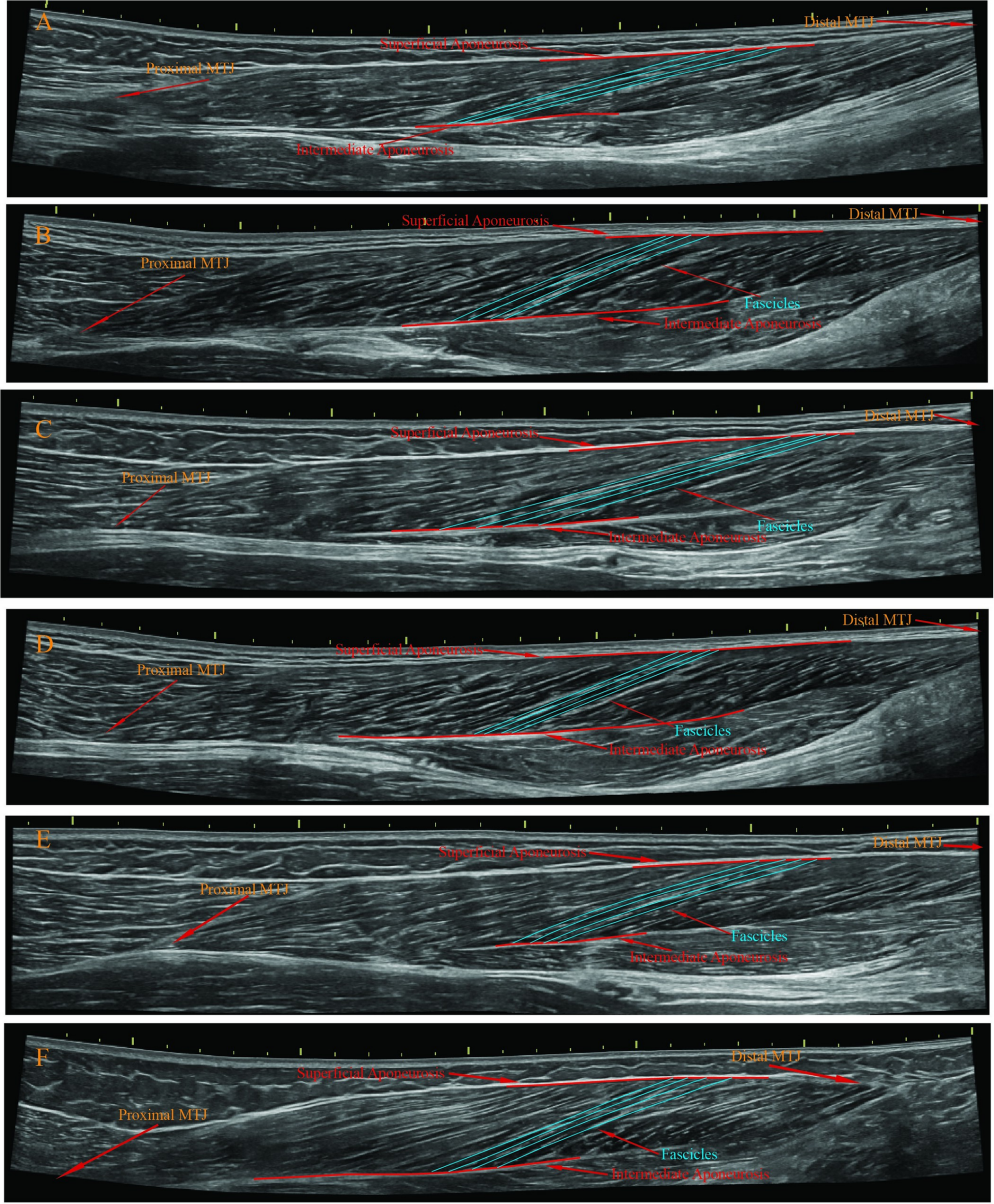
A-F: Random examples of the biceps femoris long head fascicle length measurements via panoramic ultrasound scanning in various participants.
